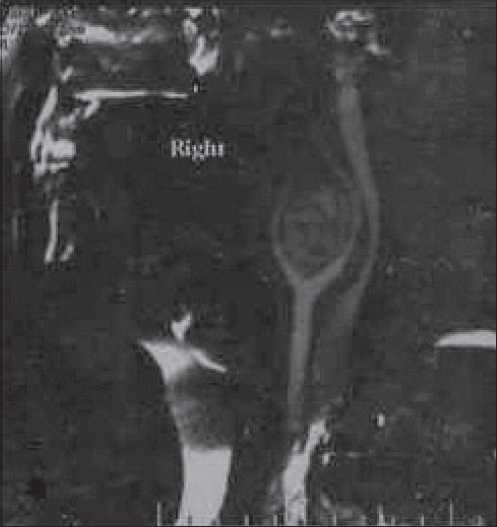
CT scan of neck reveals a tumor mass at the bifurcation of the right carotid artery
